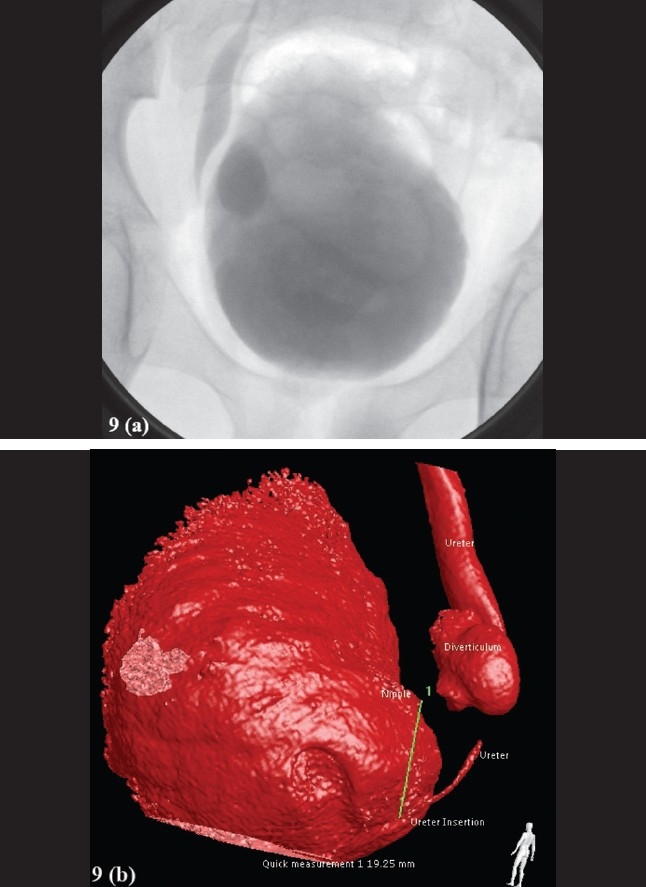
Cystogram and Rotational Fluoroscopic Tomography. Patient presented with recurrent UTI. Initial evaluation included a renal US that was normal. His VCUG demonstrated a sizable diverticulum (that increased in size after voiding) on the right side of the bladder adjacent to the UVJ(a). No VUR was identified. In order to plan the surgical approach and evaluate the need for ureteral reimplantation, a RFT study (b) was performed, the results permitted an extravesical repair. (a) Cystogram in combination with IV administration of contrast (IVP) demonstrating the diverticulum and the right ureter. (b) RFT scan demonstrating that the rightsided bladder diverticulum is not a Hutch diverticulumEvaluation of blood flow patterns of the kidney with color Doppler (to detect pyelonephritis, renal vein thrombosis and to ensure renal transplant viability - calculate the resistive index)Post void residual evaluation in patients with dysfunctional voidingEvaluation of the internal genitalia (in cases of intersex/ambiguous genitalia); in female neonates, the endocrinological stimulation by the maternal hormones causes the uterus to be visualized as a bulbous enlarged organ with a prominent endometrial stripeEvaluation of testes (structure and blood flow patterns - torsion, tumors) [Figure 8b, c, d
